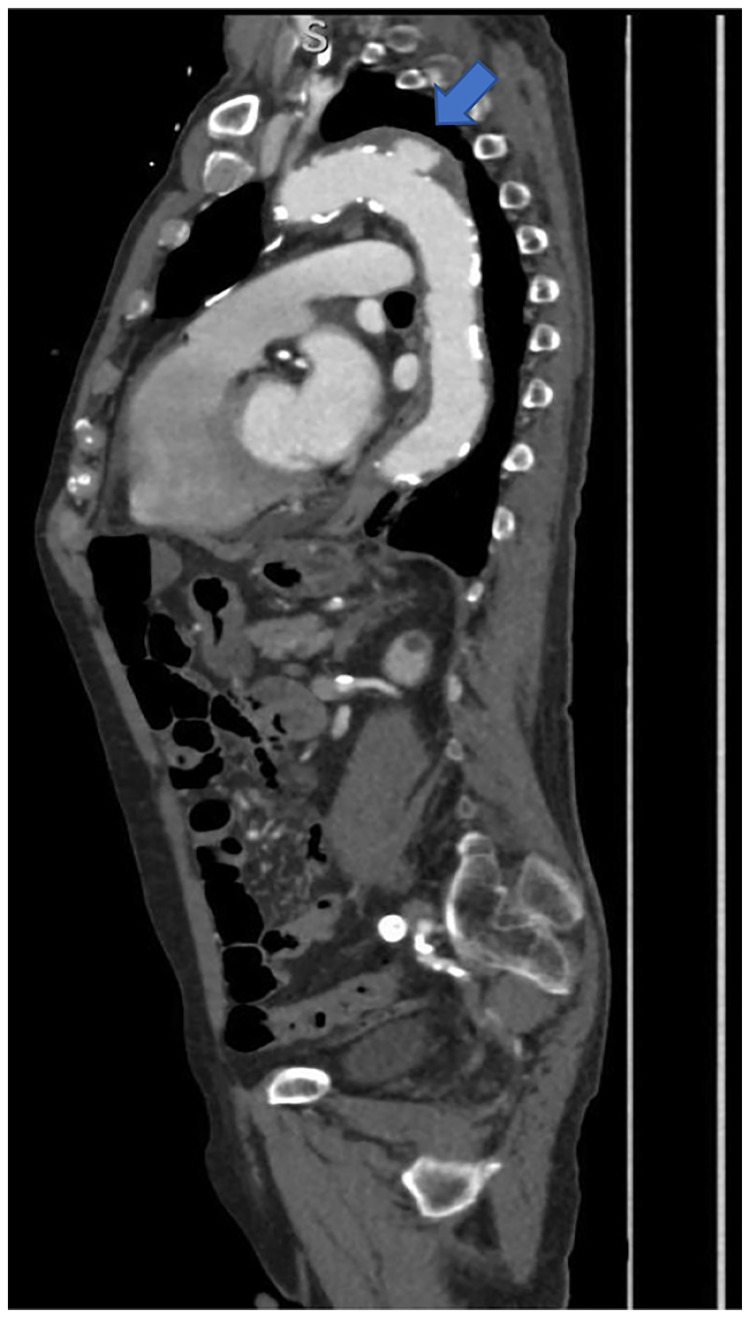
Chest computed tomography (sagittal view) showing ulcer-like projection.
